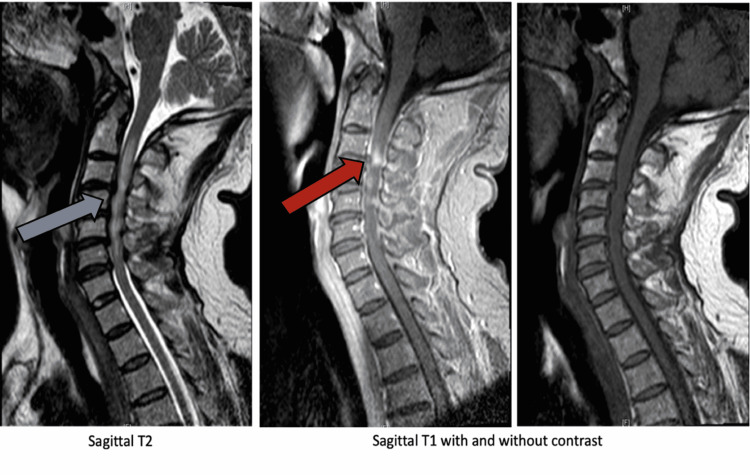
Magnetic resonance imaging of the cervical spine on presentation. Enhancement of the cord at C2 and C3 levels (red arrow) along with diffuse spinal cord edema between C2 and C7 (blue arrow).
